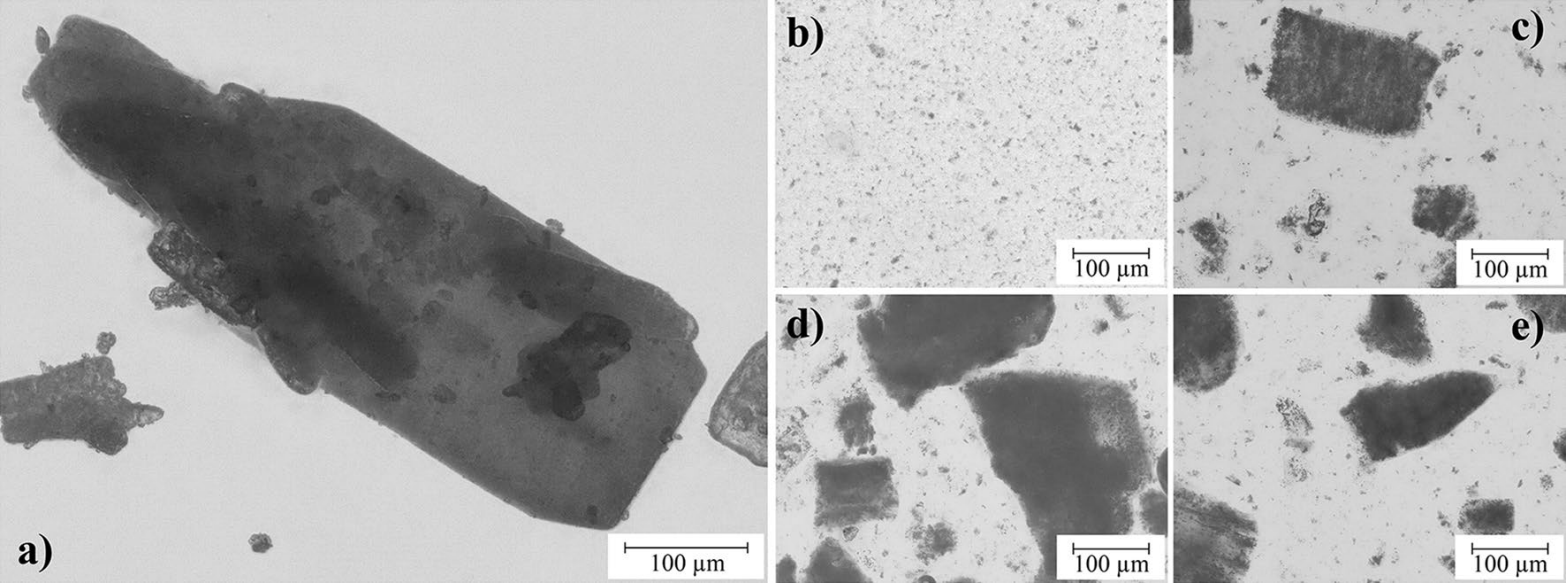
Micoscopy images of L-Arg. (a) Initial state as well as dispersed into 827 via (b) three-roll mill, (c) Thinky-Mixer, (d) ultrasonic bath and (e) ultrasonic homogeniser.
